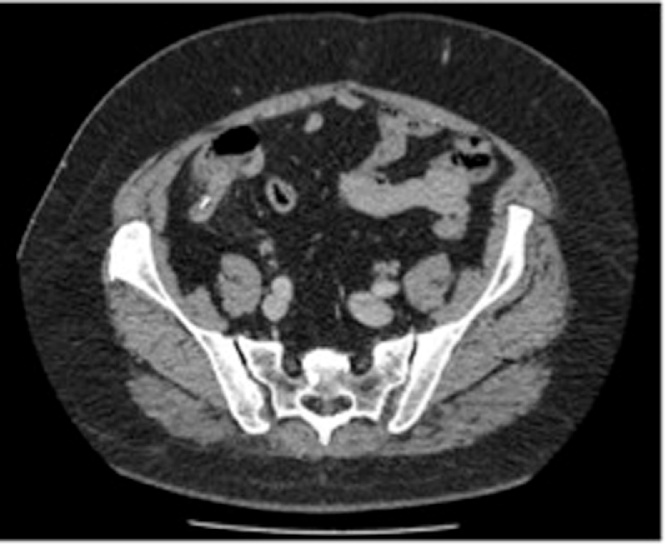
CT showing foreign body within the appendix lumen.
